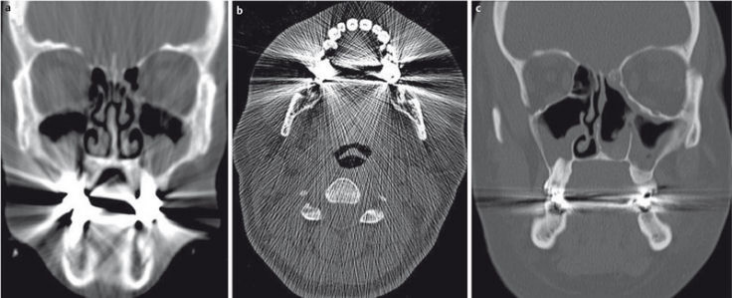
The occurrence of artifacts depends on the primary acquisition plane of the CT. a) 32-year-old female patient; CT of the paranasal sinuses acquired in primary coronal plane. Reduced image quality and diagnostic accuracy due to severe artifacts. b) 37-year-old patient with chronic rhinosinusits. CT acquired in primary axial plane is degraded by severe artifacts due to dental implants. c) Secondary reconstructed coronal plane of the same patient as in b. The artifacts are limited to the acquisition plane. Therefore, image quality and diagnostic accuracy of the paranasal sinuses are not reduced.
